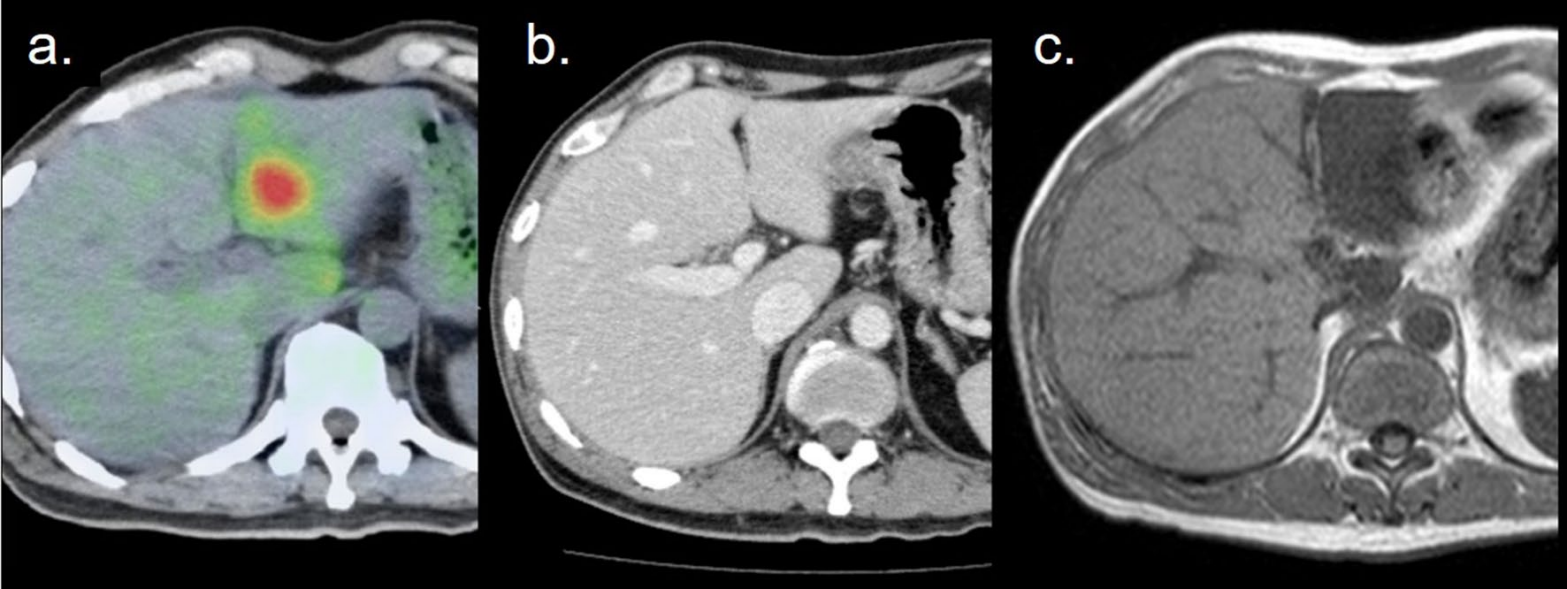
Images of a patient with testicular cancer who had undergone radiotherapy for a metastatic bone tumor at his L1 spine 2 months previously [17]. The FDG–PET/CT showed a localized hot spot in the lateral segment of the left lobe of the liver (a). Although the contrast-enhanced CT performed 10 days previously showed no abnormality (b), the MRI performed 7 days later showed a well-bordered square low signal area indicating radiation-induced hepatitis (c). (Partly cited from reference #17)
